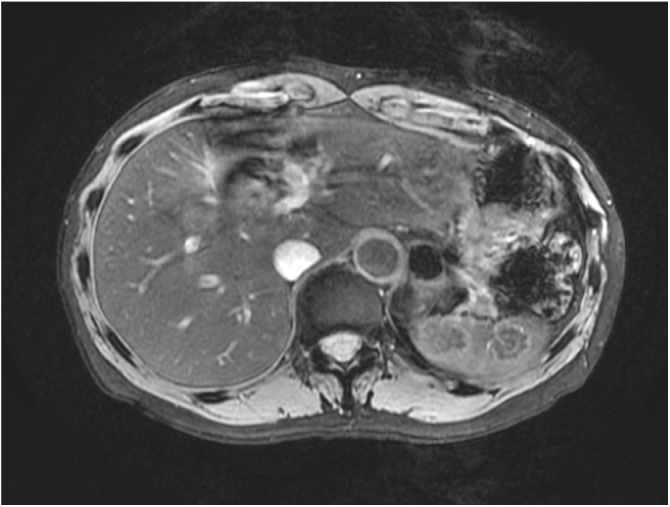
Magnetic resonance imaging of the abdomen showing hypointense nodules on T2-weighted images.
